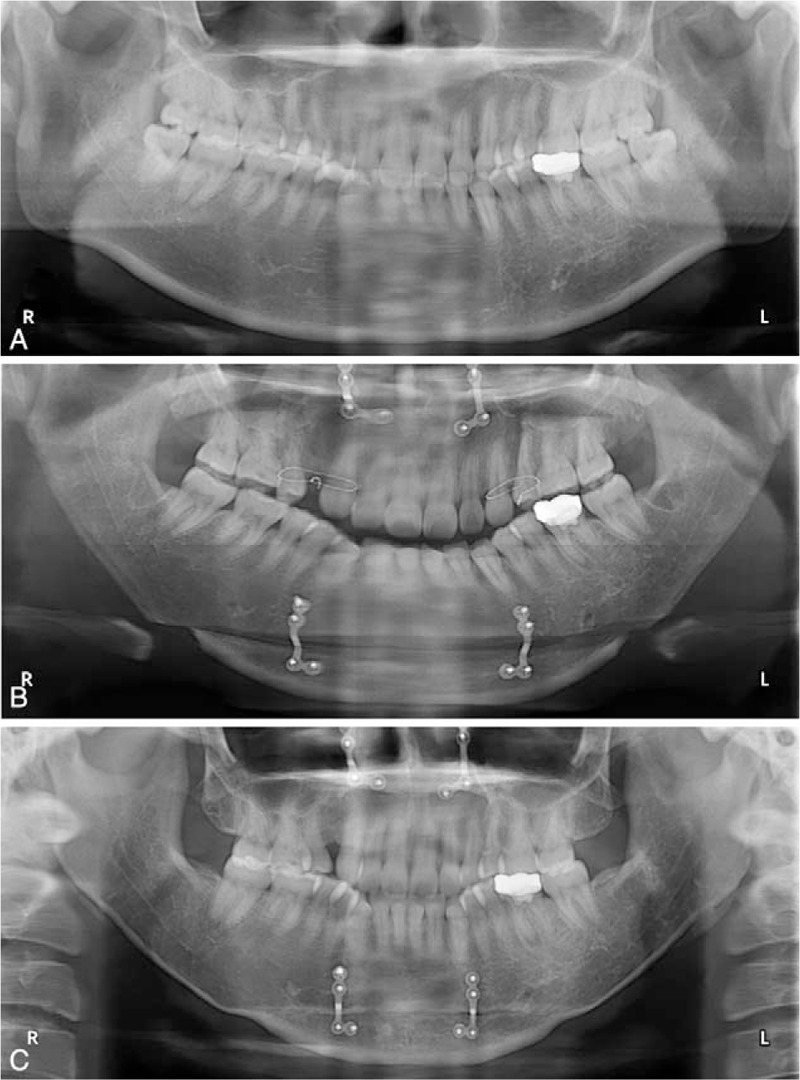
Mandibular panoramic radiographs of patient 4. Bilateral wisdom teeth were partially impacted, while the maxillary third molars had been erupted completely (A). The erupted maxillary third molars were also extracted because they would overgrow without occlusal force from the wisdom teeth (B). The 2-year post-op radiograph showed the completely healed bones (C).
